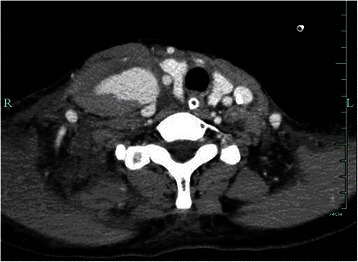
Computed Tomography Carotid Angiography showing the higher density mass (3.6*5.9 cm) of right neck connected with a branch of the subclavicular artery and compressed the right carotid artery and trachea.
